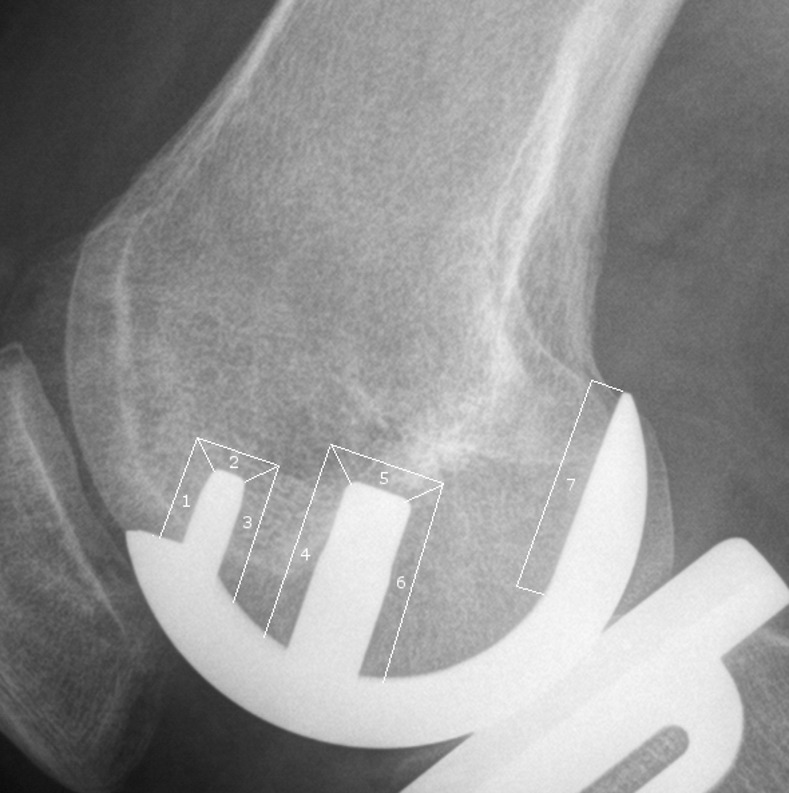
Zones of radiolucent lines on a lateral radiograph.
